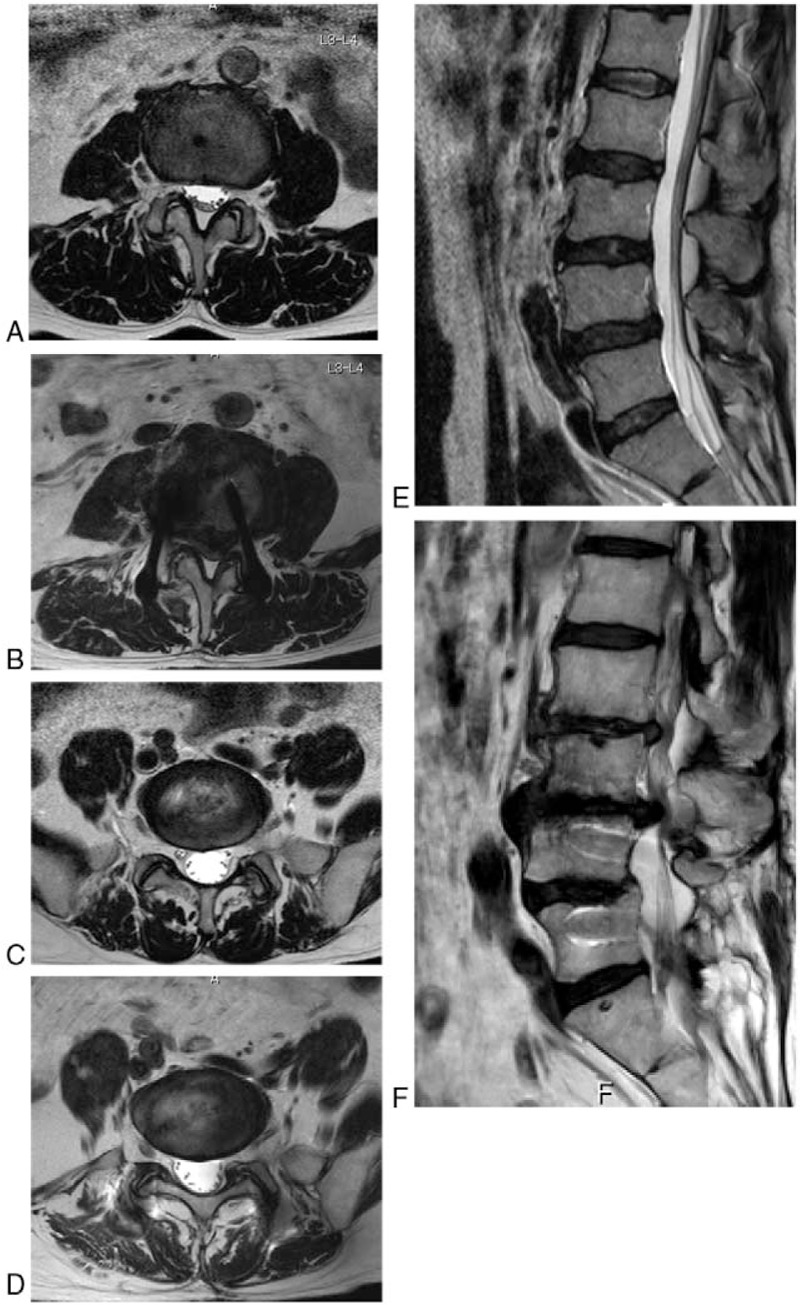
Magnetic resonance imaging scans (T2 weighted) of the patient with symptomatic adjacent segment disease at L3-L4 who underwent revision surgery 6 years after initial surgery. (A ) Axial image at L3-L4 before initial surgery. (B) Axial image at L3-L4 6 years after initial surgery. (C) Axial image at L5-S1 before initial surgery. (D) Axial image at L5-S1 6 years after initial surgery. (E) Sagittal image before initial surgery. (F) Sagittal image 6 years after initial surgery.
